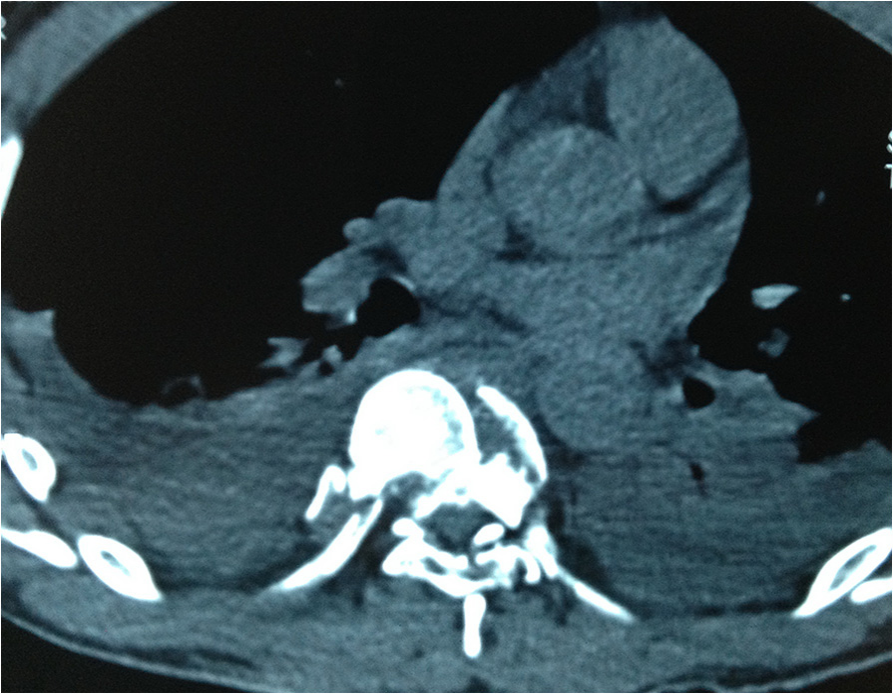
Horizontal computed tomography scans showed a large bilateral hemothorax; three vertebral levels in one cut.
